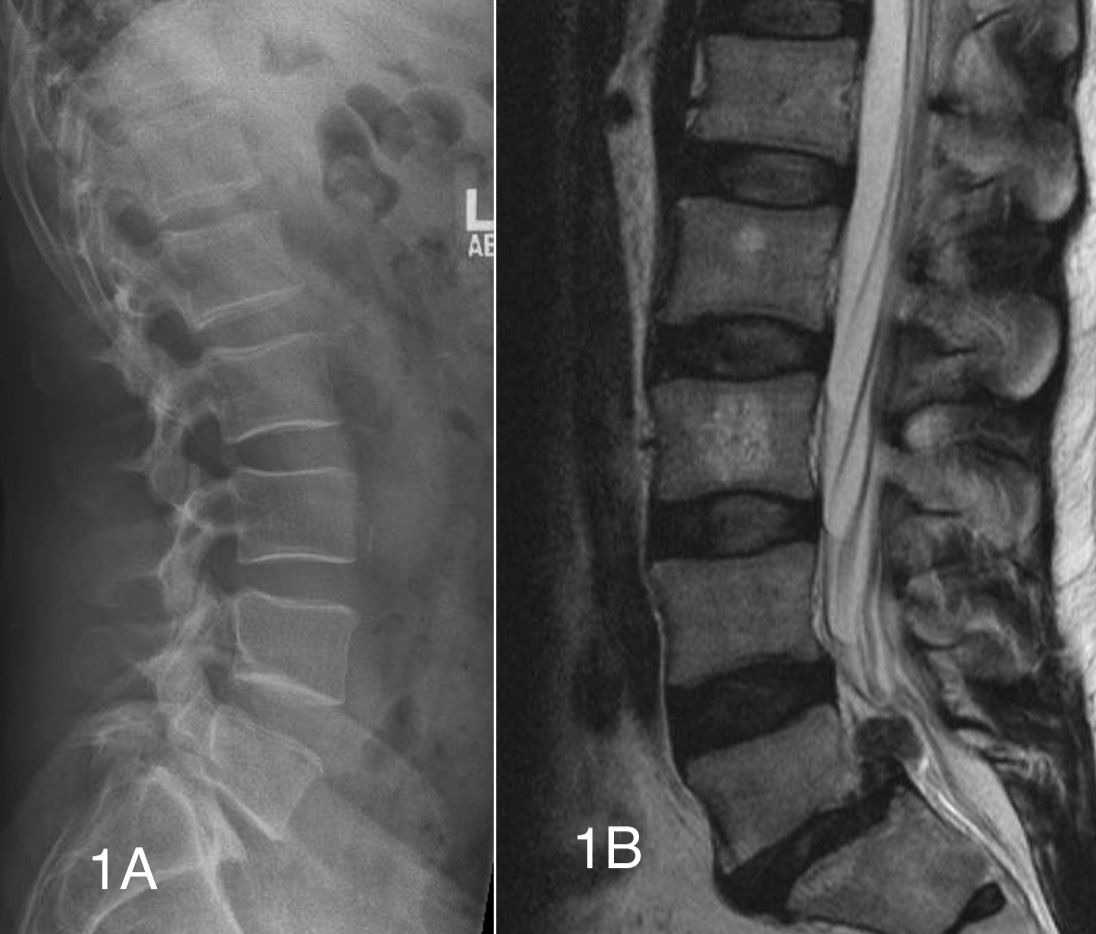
Preoperative lateral radiograph and t2 weighted sagittal MRI (Figure 1A) Lateral lumbar radiograph showing a grade I L5-S1 spondylolisthesis. (Figure 1B) T2 sagittal MRI reveals a large L5-S1 disc herniation in the setting of grade I L5-S1 spondylolisthesis.
